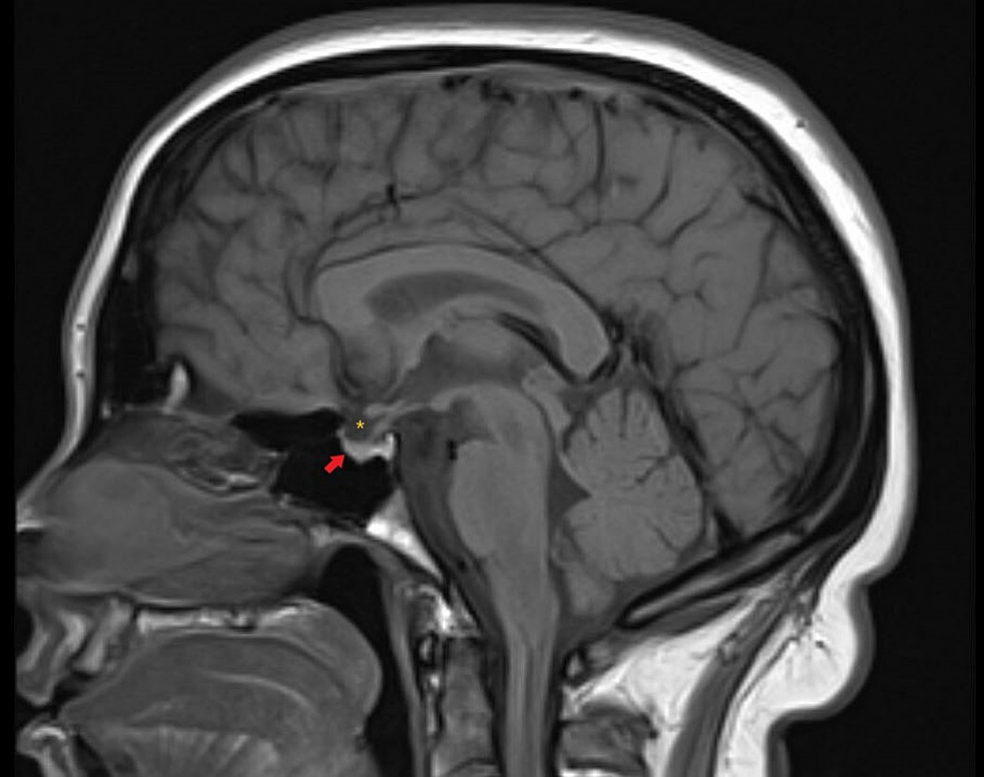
Magnetic resonance imaging brain: Sagittal T1 weighted image showing a flattened pituitary gland (red arrow) suggestive of partially empty sella appearance and cerebrospinal fluid in the sella (yellow asterisk)
